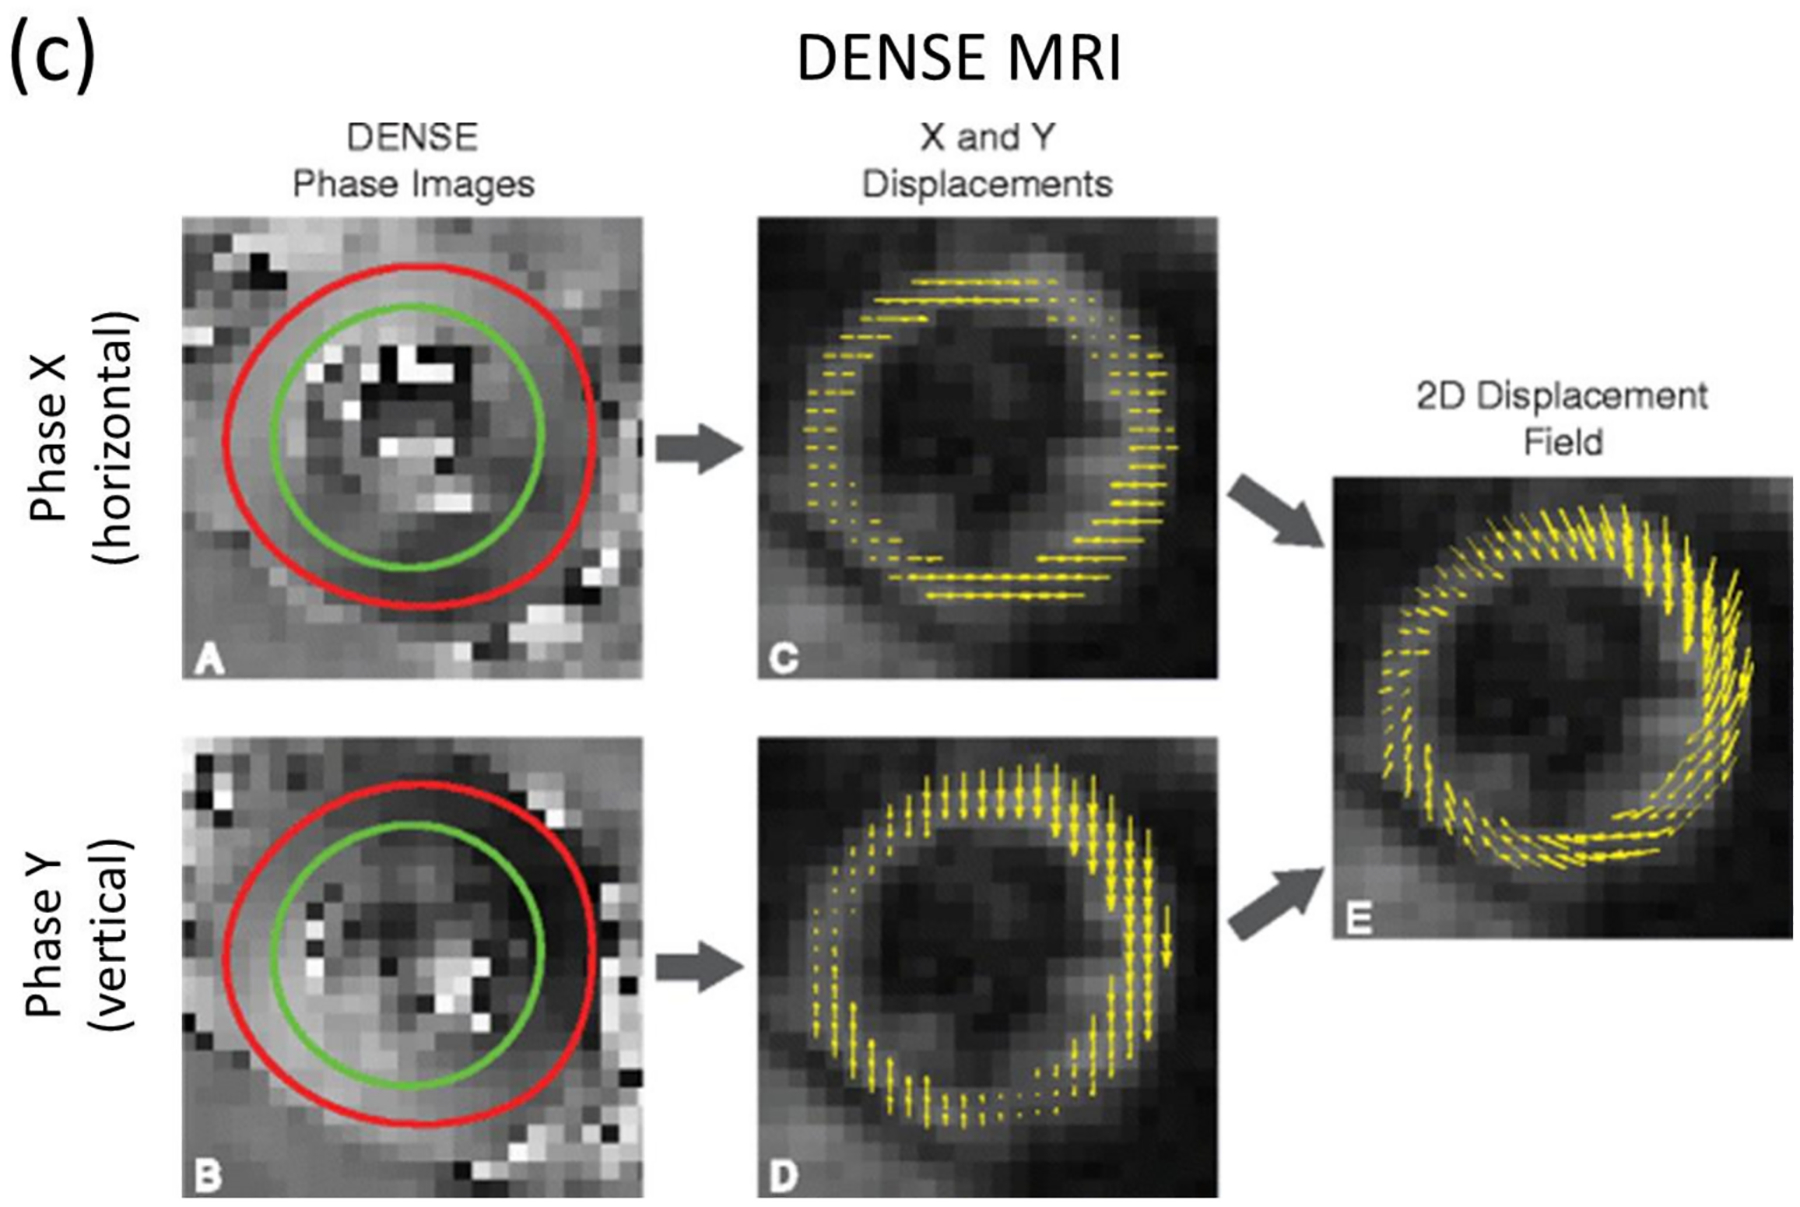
Examples of image-based kinematics of the left ventricular myocardium with (a) Speckle tracking ultrasound (Reprinted/adapted with permission from Ref. [169]. 2020, Alessandra M. Ferraro et al.; open access) (Left) Dots indicate speckle-kernel location and identification, (right) green lines indicate trajectory through the cardiac cycle. (b) Tissue tagging (Reprinted/adapted with permission from Ref. [170]. 2012, The Radiological Society of North America). Tissue tagging estimates kinematics by tag-to-tag tracking from diastole to systole. Transversal (green lines) and diagonal (red lines) tag-to-tag dimensions are measured at diastole (left column) and systole (middle column), their difference can be used to measure displacement and deformation (red lines in right column). (c) DENSE MRI (Reprinted/adapted with permission from Ref. [171]. 2015, Wehner et al.; licensee BioMed Central, open access). DENSE MRI resolves pixel-wise displacements by processing phase data for each direction. Red and green contours represent segmented luminal and adventitial boundaries, yellow arrows represent the phase-encoded displacement.
